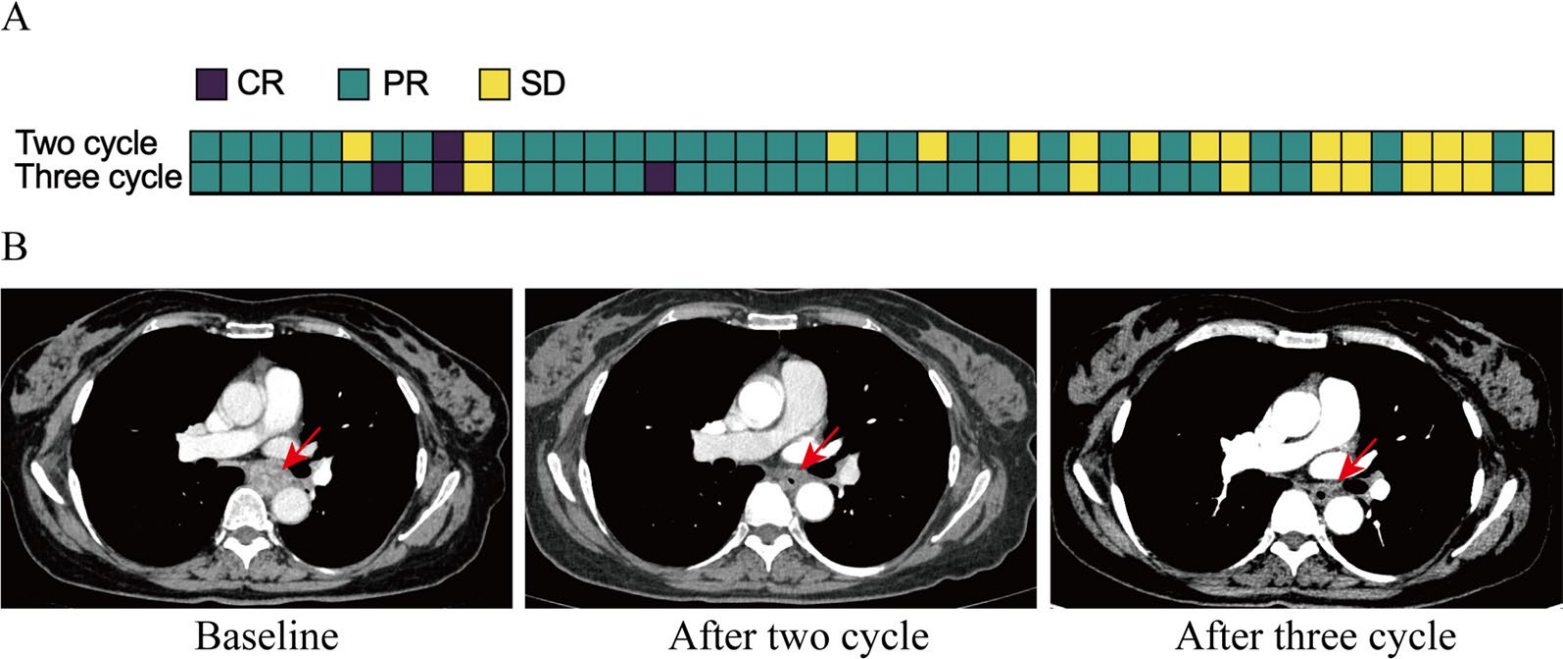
Clinical response to neoadjuvant immunochemotherapy. A Response assessment with computerized tomography (CT) before the initiation of treatment and after two and three cycles of neoadjuvant immunochemotherapy. B Representative CT scans of patient who achieved partial response (PR) following the second cycle and further achieved complete response (CR) after the third cycle treatment. Red arrow indicates the local tumor lesion. CR: complete response; PR: partial response; SD: stable disease
